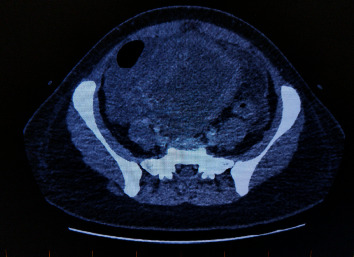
CT report of the abdomen and pelvis. Large pelvic mass which may be ovarian in nature. The fluid content of this cystic lesion is 24 HU in density. It has a thick irregular wall. There is generalized ascites and peritoneal induration.
